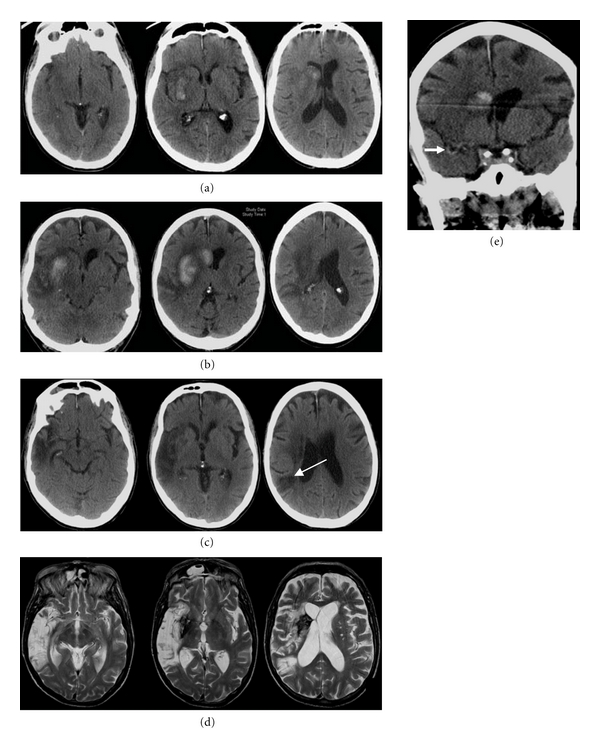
69-year-old man with atrial fibrillation on warfarin, he presented with a 2-day history of left hemiparesis and neglect. (a) Initial axial unenhanced CT images show hemorrhage in the right basal ganglia, involving the right caudate head, unusual for ICH. Stage IIH d1 [1]. Warfarin was stopped and anticoagulation was reversed. (b) Axial unenhanced CT images 10 days later show further extension of hematoma with the surrounding hypodensity extending out to the cortex. (c) Axial unenhanced CT images 1 month after stroke show an extensive area of hypoattenuation in the right MCA territory. There is also an area of low attenuation more posteriorly (thin arrow), not evident on the previous CT and separate from the initial stroke, raising the possibility of a new subacute infarct since the first stroke. (d) Axial T2-weighted MR images 3 months after stroke show the posterior temporal infarct evident on the last CT scan more clearly, suggestive of bland infarction in that region. The cessation of warfarin after the first stroke probably contributed to this cardioembolic stroke. (e) On reviewing the initial CT images, a dense right middle cerebral artery sign is present on the coronal view (but not axial), further suggesting the first stroke is a hemorrhagic infarct rather than a hemorrhagic stroke.
